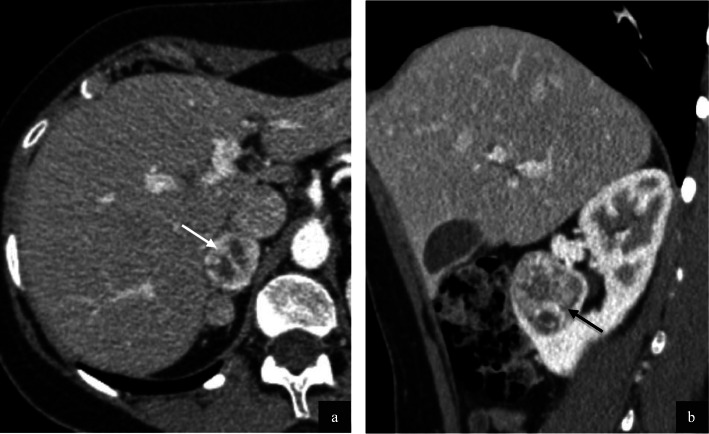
Adrenal (a) and renal (b) metastases in a 21 year old female with alveolar soft part sarcoma. Portal venous phase contrast enhanced axial (a) and sagittal (b) CT demonstrates a lobulated, hyperenhancing right adrenal metastasis (a, white arrow) with central necrosis. The enhancing, right lower pole renal metastasis (b, black arrow) shows minimal necrosis
